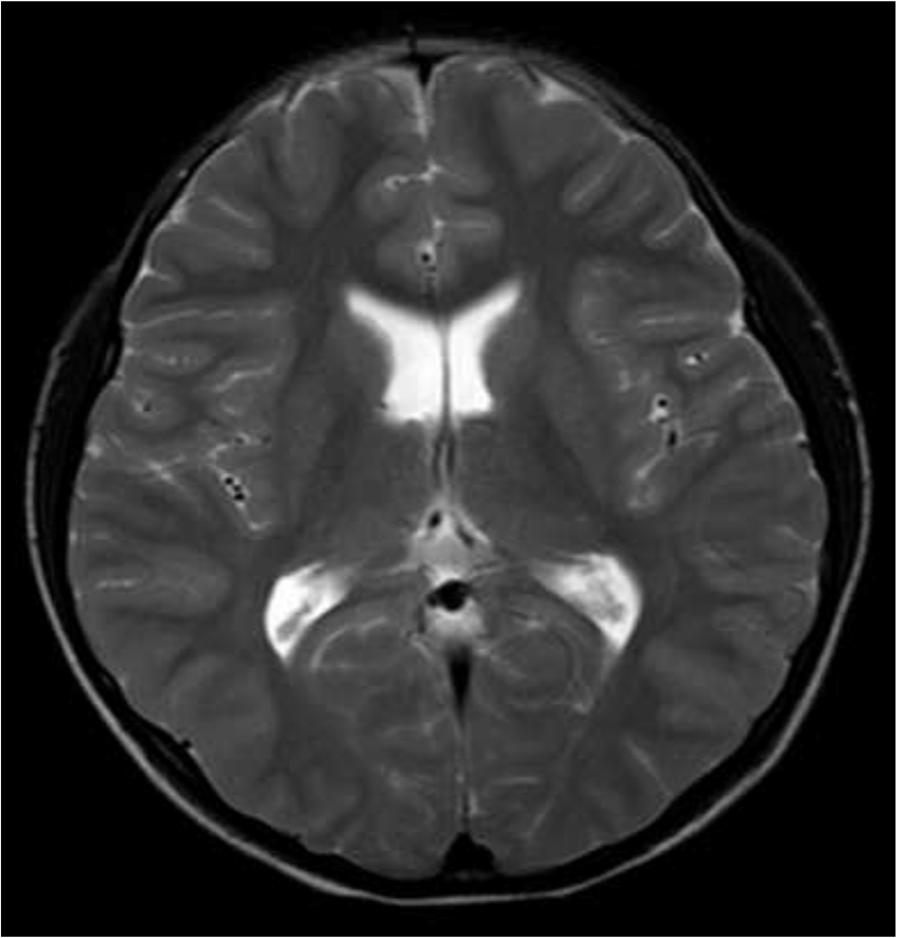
Cranial magnetic resonance imaging (MRI) was normal
